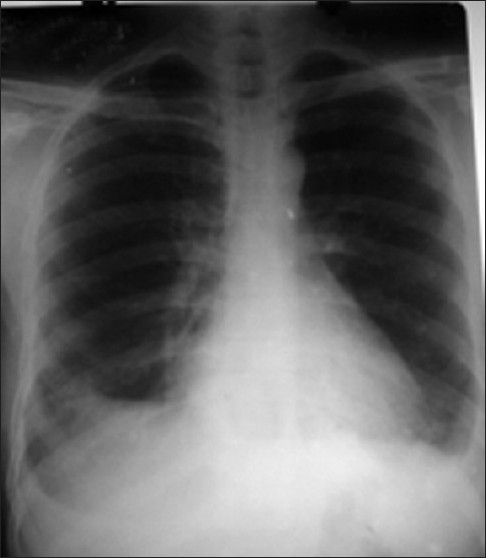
The chest X-ray after he presented to us showed homogenous opacities with bilateral pleural effusion
